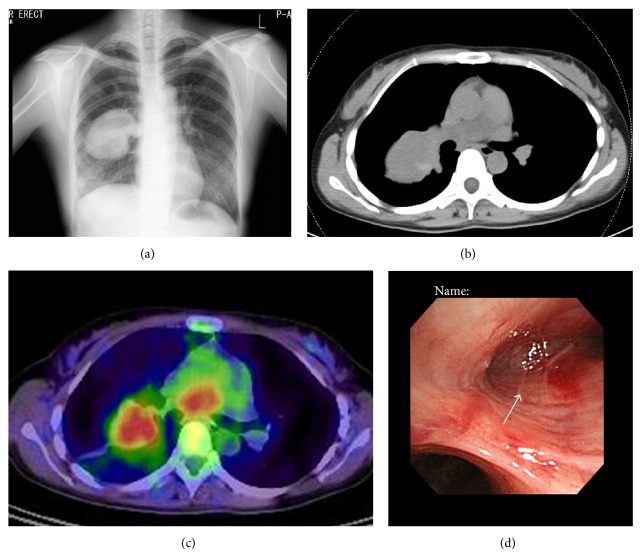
(a) Chest radiography showing a mass in the right middle lung field. (b) Noncontrast CT showing a mass approximately 8 centimeters in diameter with relatively smooth margins in the right lung segment 6. (c) Fluorodeoxyglucose-positron emission tomography showing accumulation in the right lung mass and in the lesion in the mediastinum. (d) On bronchoscopy, a whitish tumorous lesion was seen obstructing right B6 (arrow).
